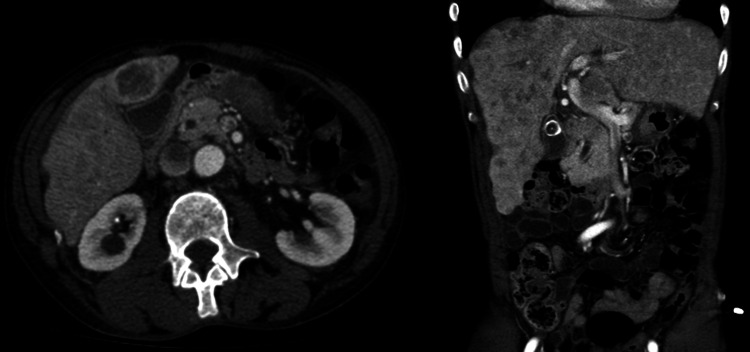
Axial and coronal images from a contrast-enhanced CT scan of the abdomen. Multiple hypoattenuating foci throughout the liver compatible with multiple hepatomas before TACE can be seen. TACE, transarterial chemoembolization
